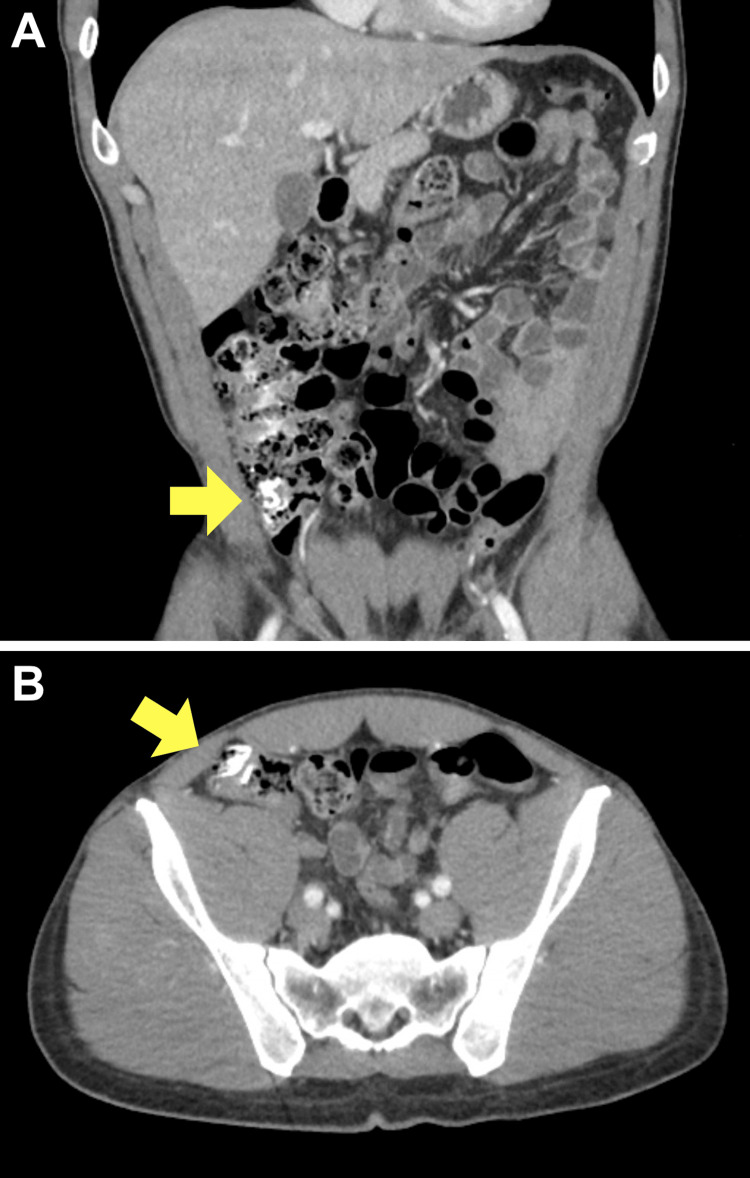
Abdominal computed tomography scan of a 41-year-old male with acute appendicitis Coronal (A) and axial (B) images of the dilated appendix with thickened, enhancing walls and periappendiceal fat infiltration (arrows)
